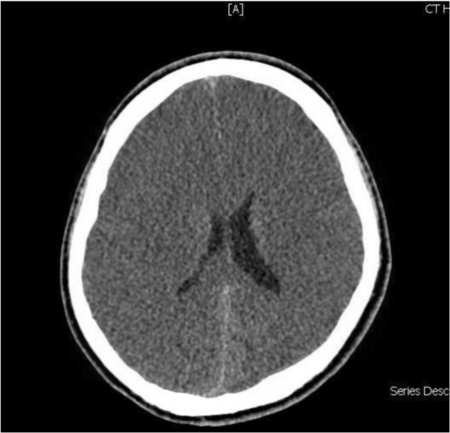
Computed tomography brain scan without contrast showing a generalized loss of the supratentorial grey-white matter differentiation with effacement of sulci indicating increasing degree of diffuse cerebral edema. Asymmetry of lateral ventricles is seen with slit-like appearance of anterior horns. An effacement of the basal cisterns can be noted as well
